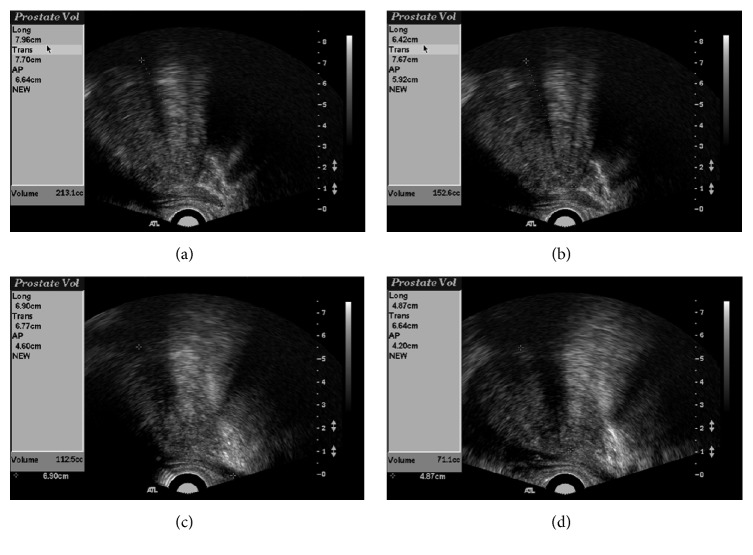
Transrectal ultrasound (US) images before embolization and at follow-up after prostatic artery embolization (PAE) (the same case as shown in Figure 1). Total volume (a and c) and transition zone volume (b and d) were obtained. US images obtained before PAE (a and b) and 24 months after PAE (c and d) showing marked reduction in prostatic volume.
